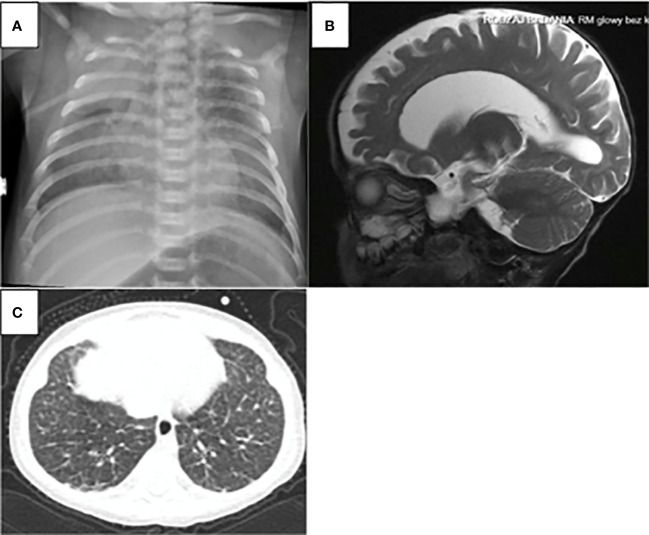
ADA-SCID sypmtoms: (A) Chest X-ray: scapular spurring with flaring and cupping of rid ends in the patient 6. (B) atrophic changes in the CNS were found in the brain magnetic in the patient 3 (C) computed tomography of the chest as ground-glass opacities with interlobular and intralobular interstitial thickening in patient 5.
